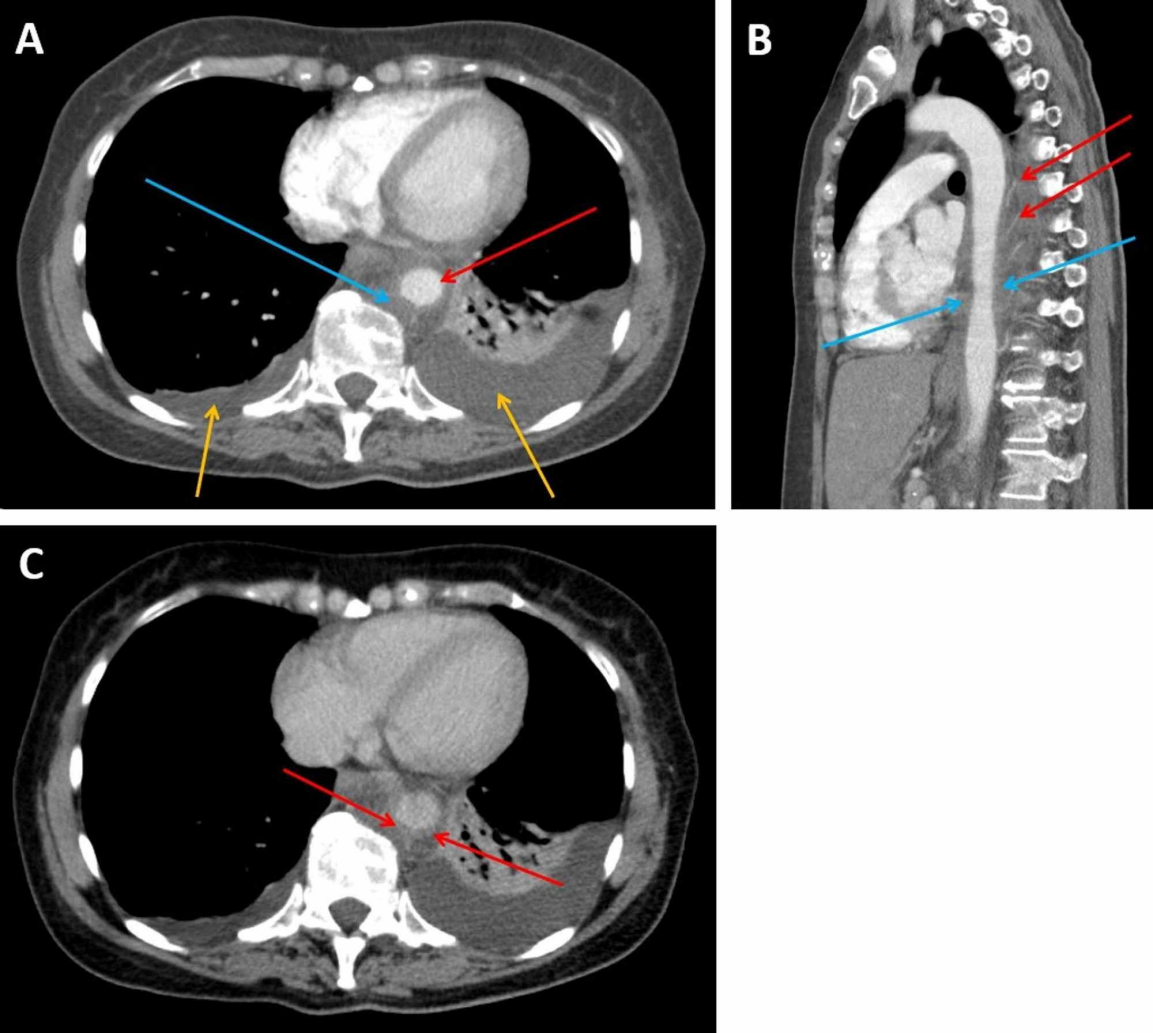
CT imaging of the thorax, abdomen and pelvis performed on the fourth day of admission (A: transverse plane, arterial phase; B: sagittal plane, arterial phase; C: transverse plane, portal venous phase) A: This arterial phase image shows expansion and opacification of the posterior mediastinal fat due to haemorrhage. The aorta (red arrow) has been displaced from the vertebra and is compressed by a peri-aortic haematoma (blue arrow). The lumen is now narrowed measuring 18mm x 16mm. Bilateral pleural effusions are also present (yellow arrows). B: This arterial phase image demonstrates ectatic narrowing of the aorta (blue arrows) with circumferential hyperdensity indicating peri-aortic haematoma and splaying of the intercostal arteries reflecting mass effect (red arrows). C: This image, taken in the portal venous phase, better demonstrates the aortic mural thickening (red arrows).
